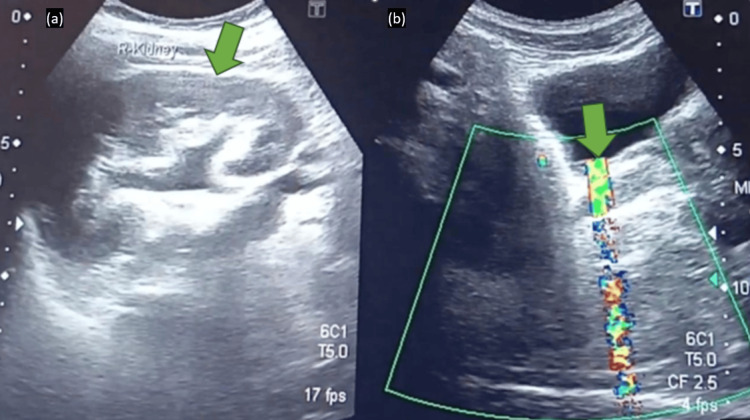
Ultrasound Showing Mild Right-Sided Hydroureteronephrosis and Distal Ureteric Echogenic Focus (a) Ultrasound image showing mild right-sided hydroureteronephrosis (green arrow). (b) Ultrasound image demonstrating a distal ureteric echogenic focus at the vesico-ureteric junction (green arrow) with posterior acoustic shadowing and color-Doppler twinkling artefact, consistent with an impacted calculus.
